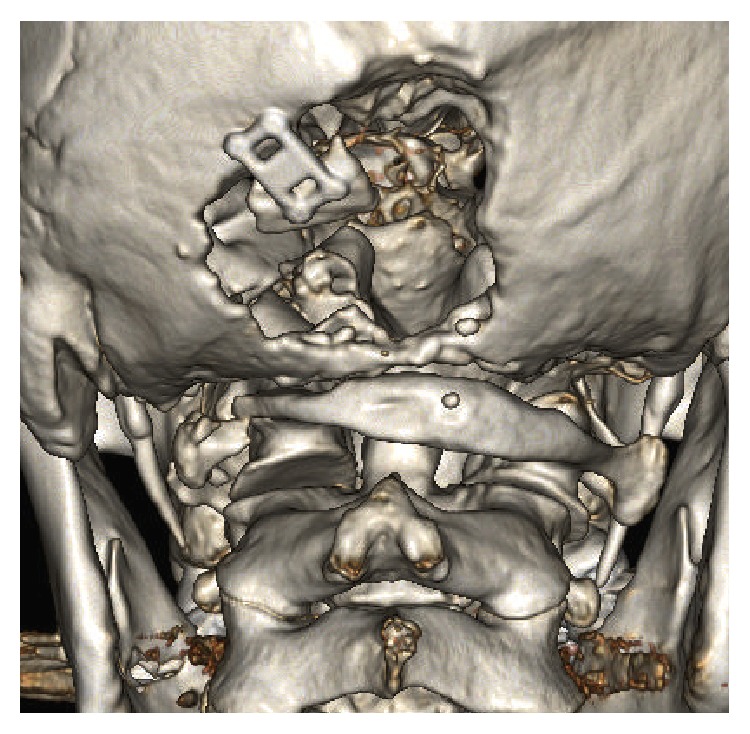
Computed tomography of the head and neck when the patient was referred to our hospital. Defects are observed in the left side and midline of the occipital bone.
